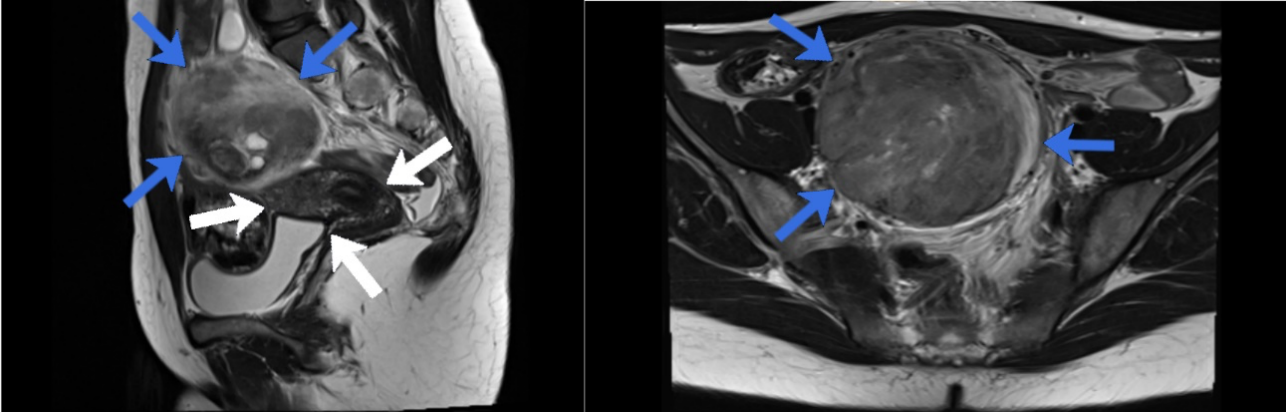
MRI images in T2W: A: MRI in sagittal view showing the uterus (white arrows) and the schwannoma (blue arrows). B: MRI in axial view showing the schwannoma (blue arrows).
